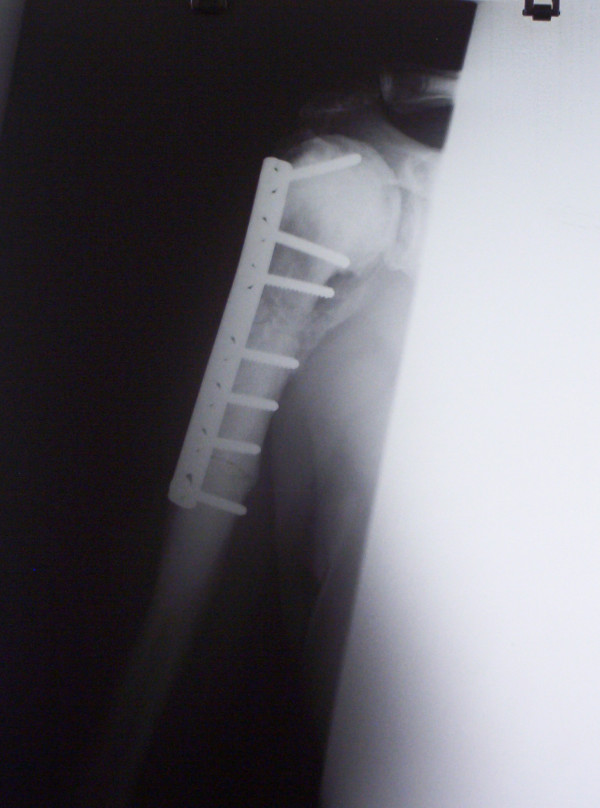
Postoperative X-ray image of fracture after 6 months with healing and good reduction.
